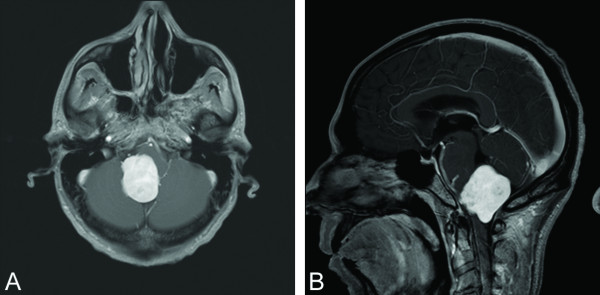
Contrast-enhanced T1-weighted axial (A) and sagittal (B) magnetic resonance imaging scans showing a homogenous mass located in the fourth ventricle.
